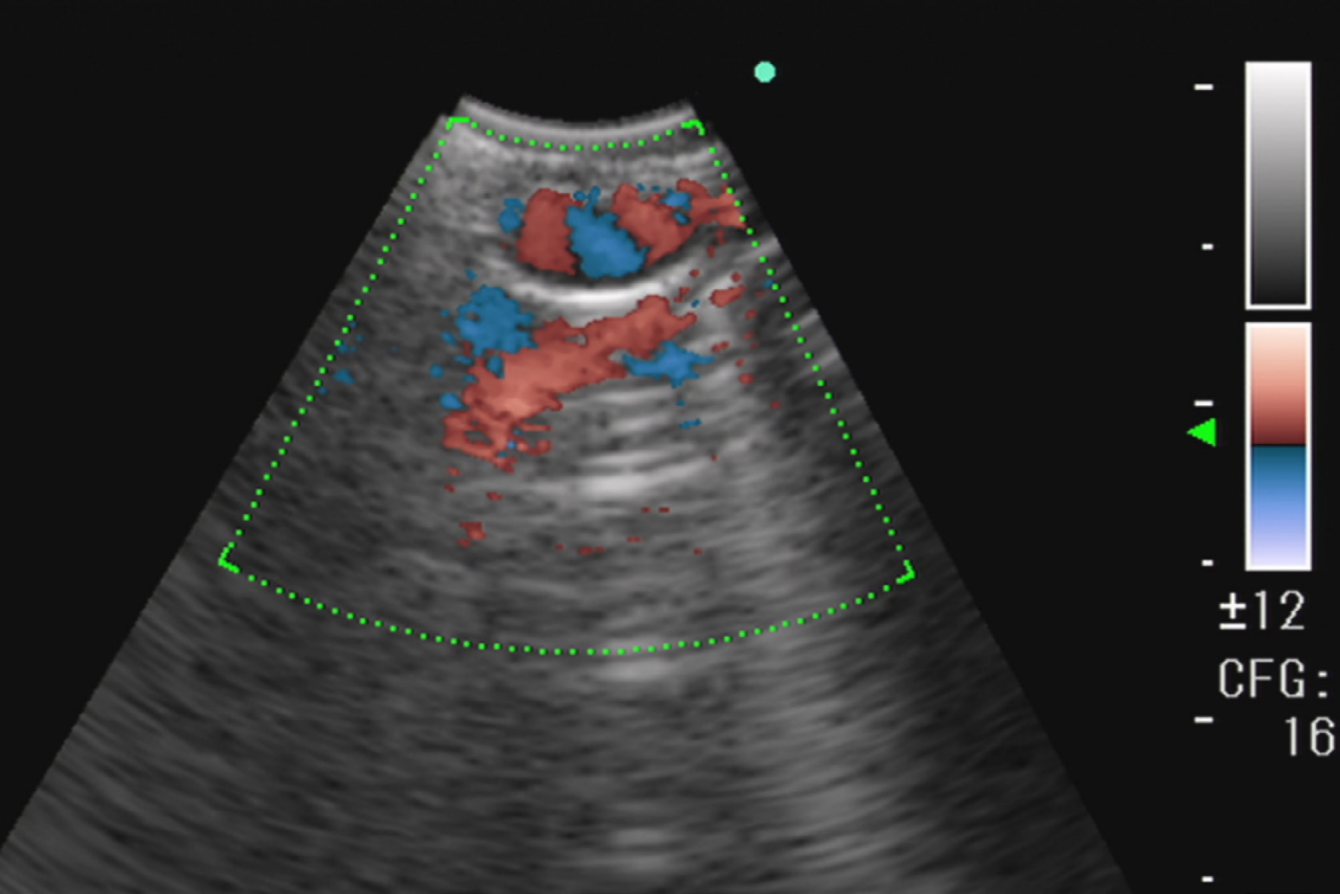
Convex‐probe endobronchial ultrasound. Abnormal blood flow beneath the polypoid lesion was seen.
